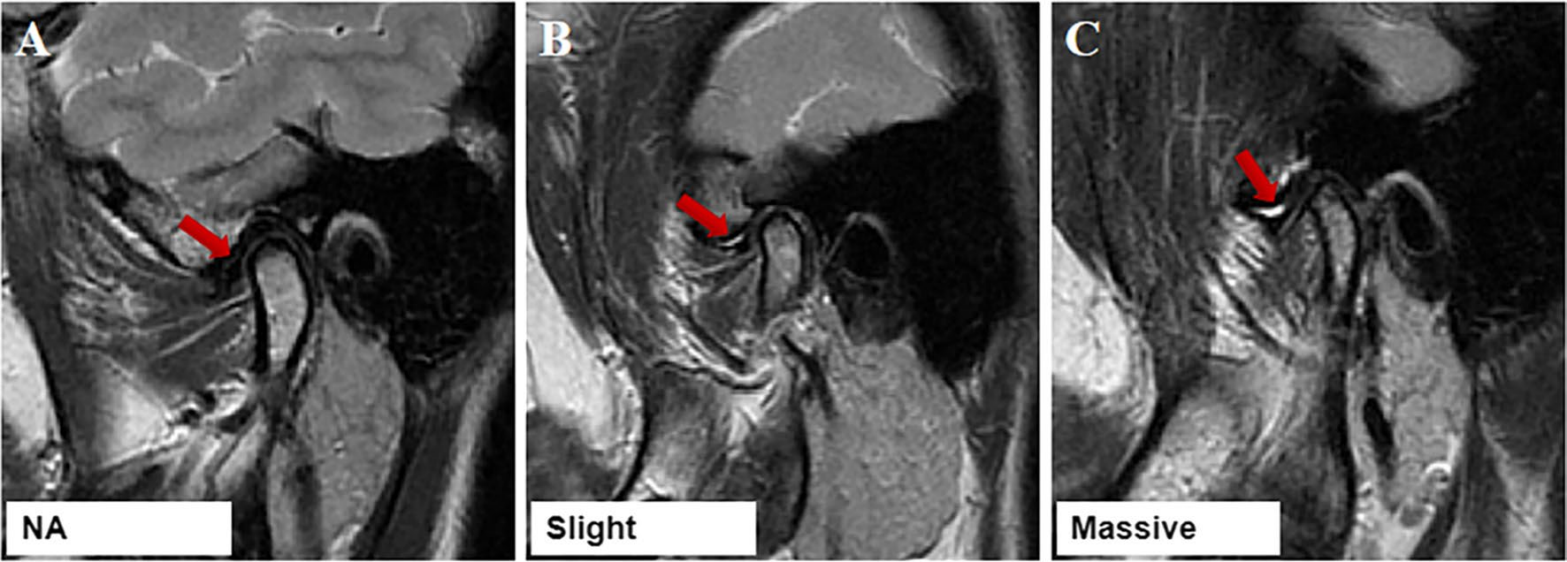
MRI images show joint effusion where the arrow indicates the effusion. A NA = no joint effusion; B slight joint effusion; C massive joint effusion. Where: NA: normal
